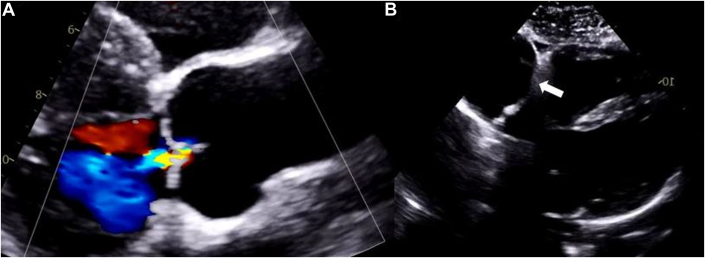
Transthoracic Echocardiography Echocardiogram revealed an enlarged sinus of Valsalva with mild aortic regurgitation (A) and no aortic stenosis. (B) A cyst was detected adjacent to the right atrioventricular groove (arrow).
